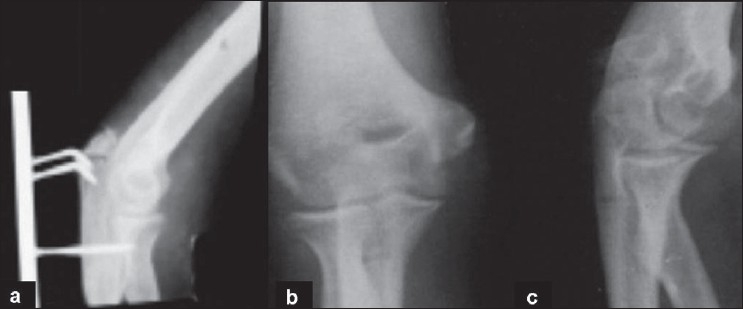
(a) X-ray of the elbow (lateral view) showing implant cut out. X-ray (anteroposterior (b) and lateral views (c)) of the same patient showing union of fracture in elongation.
